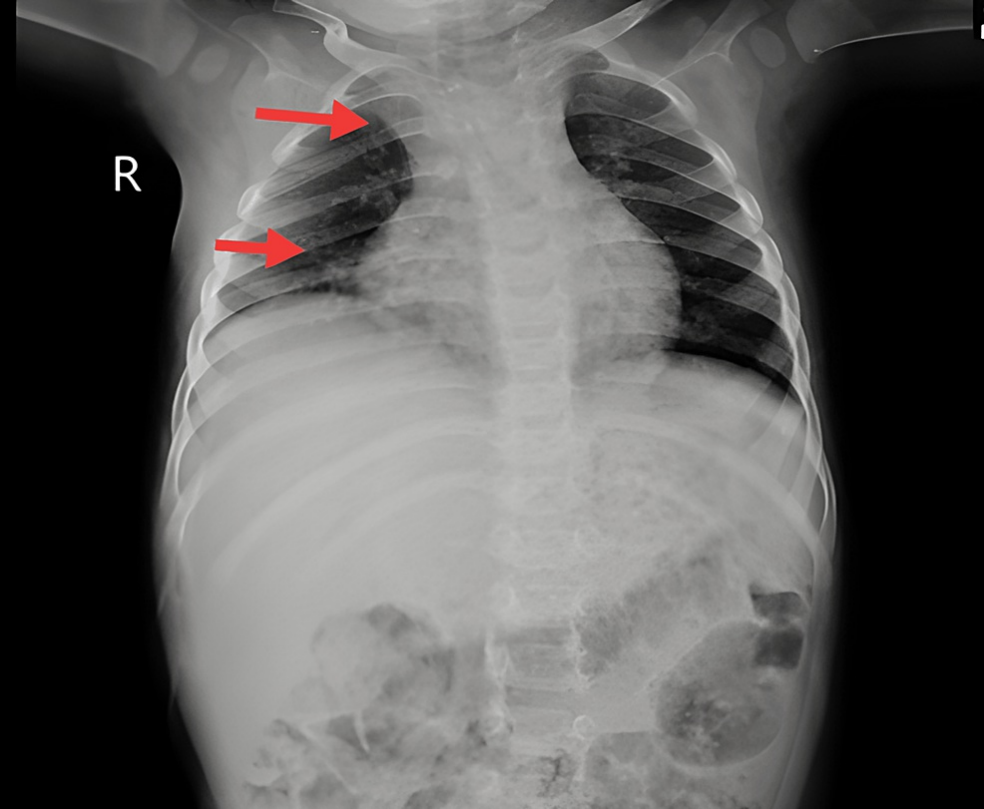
Chest X-ray of the patient featured broncho-pulmonary markings evident of infection and widening of mediastinum.
